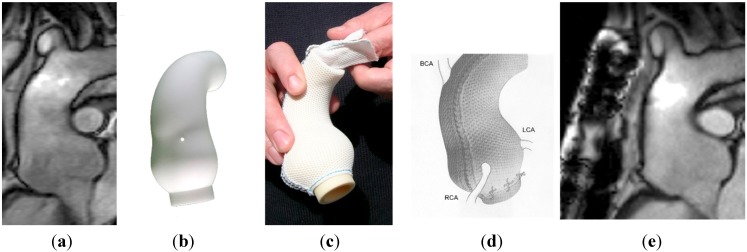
(a) The aorta before surgery. (b) The model on the computer screen after computer assisted design (CAD) modelling. (c) The soft macroporous mesh with 0.7 mm pore size mesh. (d) A depiction of the mesh surrounding the aorta and tethered to the ventricle with the coronary arteries emerging. (e) The MRI stable after 10 years.
